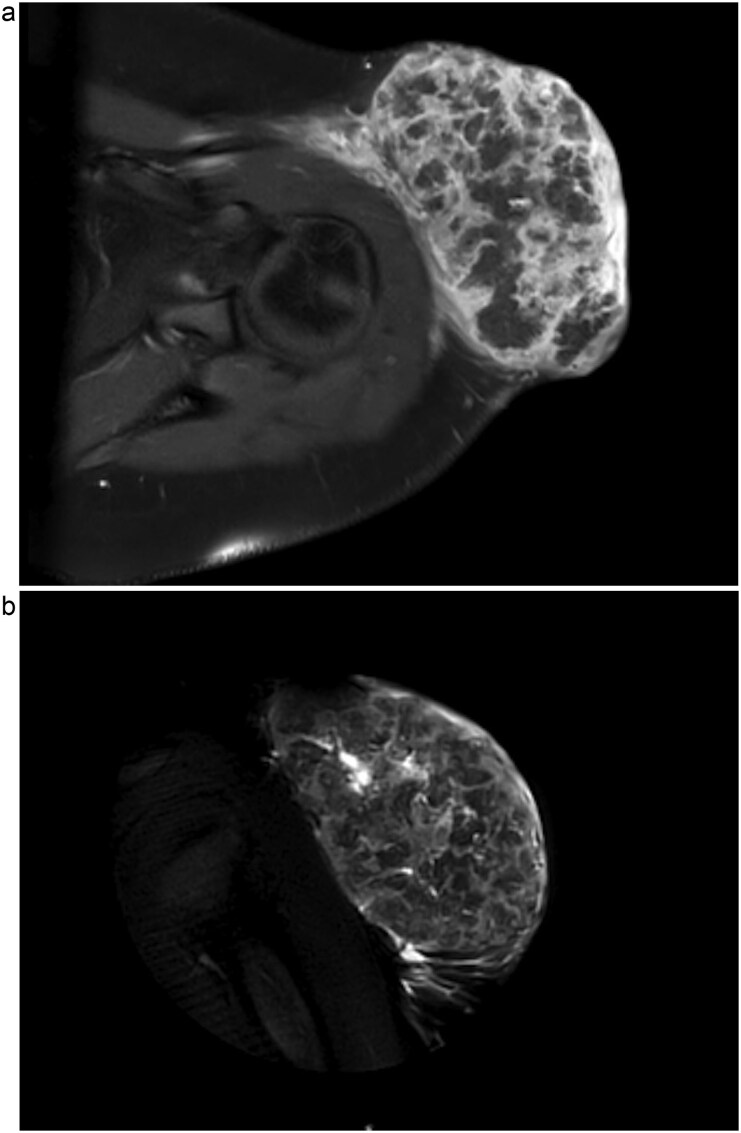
1.5 Tesla MRI with axial view (a) and coronal view (b) showing a 10.8 × 6.7 × 7.4 cm left anterolateral shoulder soft tissue mass. This is suspicious for malignancy, most likely sarcoma, due to lack of a soft tissue plane between the tumour and the deep deltoid musculature as well as enlarged axillary lymph nodes (not shown).
